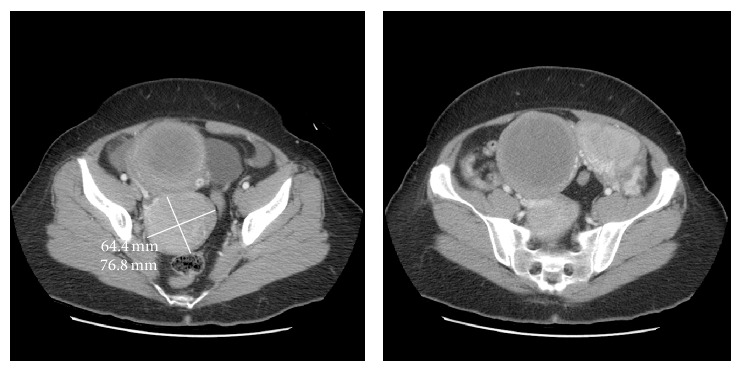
Representative computed tomography (CT) images of the abdomen and pelvis showing uLMS as a large heterogeneous uterine tumor.
